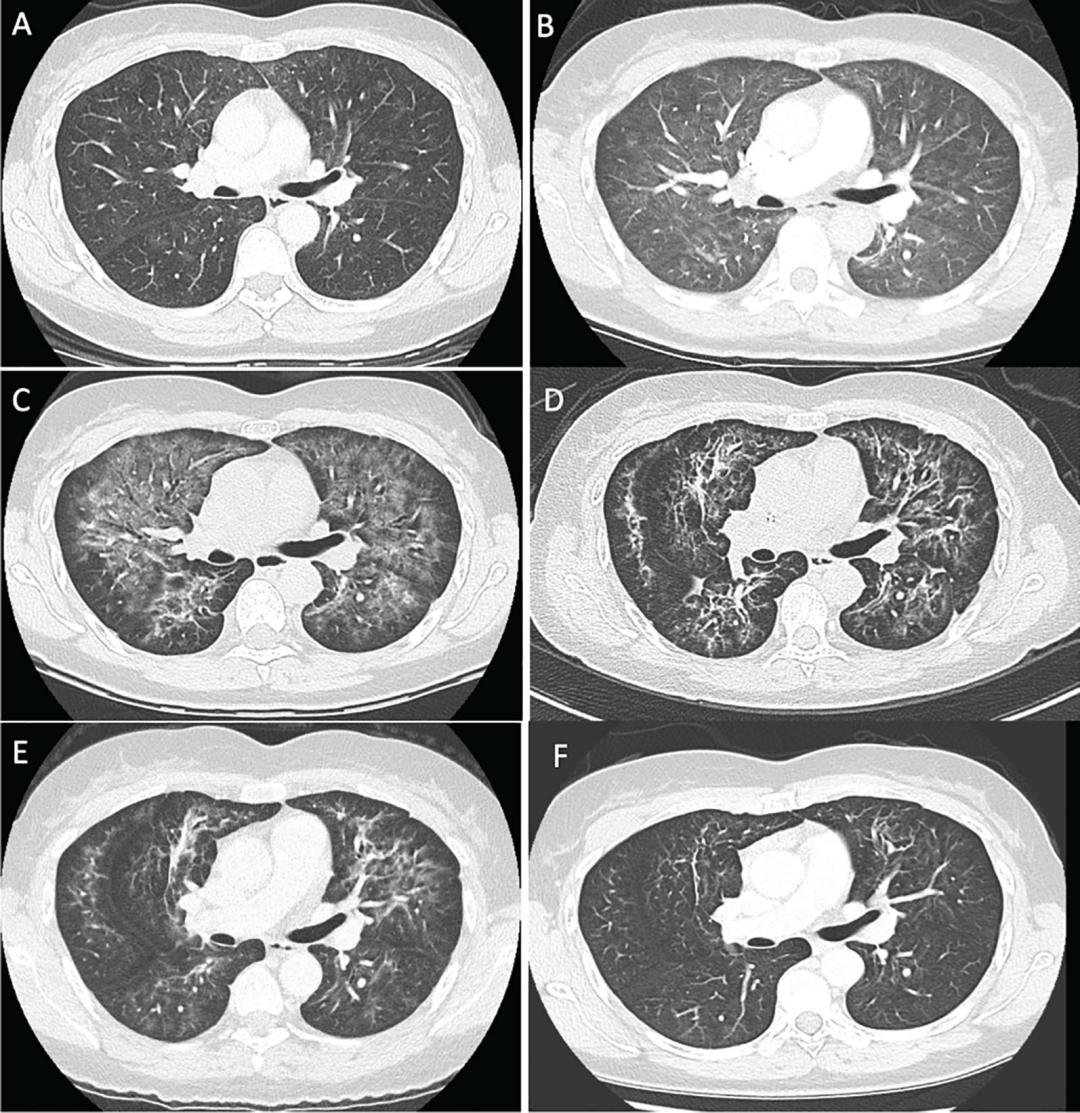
CT chest appearances at (A) baseline prior to immunosuppression, (B) following 2 weeks of oral corticosteroid therapy, (C) after intravenous methylprednisolone and mycophenolate, (D) after eATG, (E) 6 weeks after commencing eATG, and (F) at 9 months.
